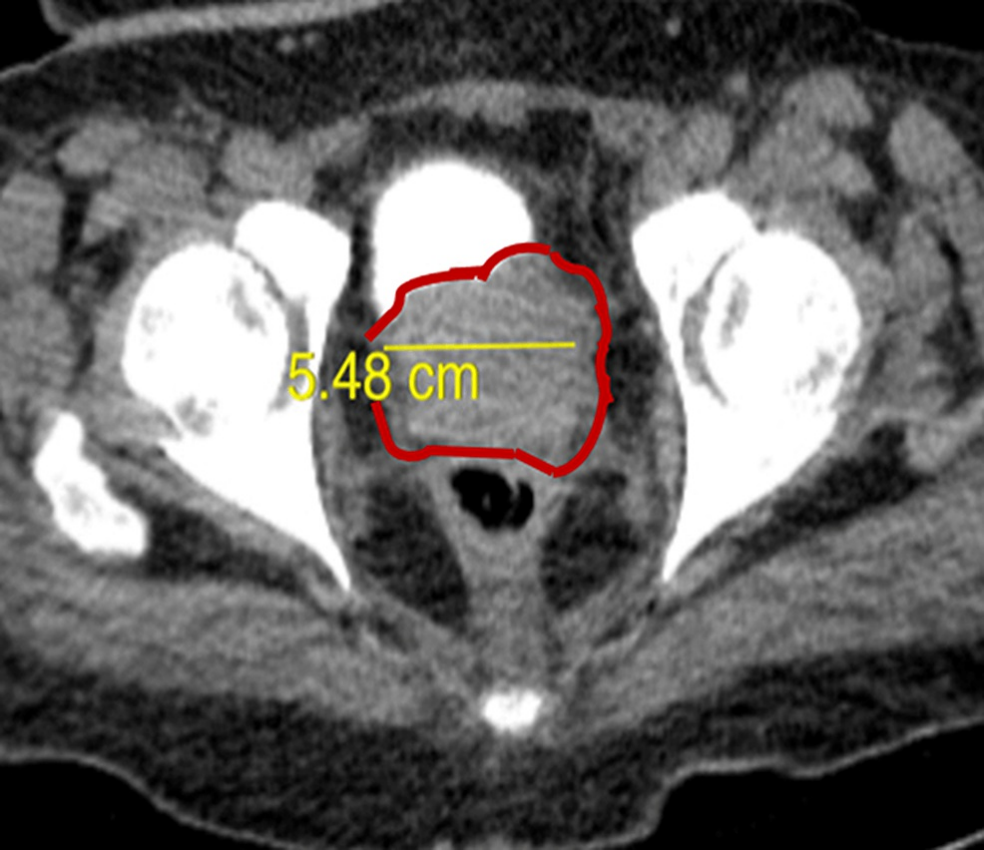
Axial CT scan of the pelvis. Pelvic CT scan showing enlarged prostate measuring 5.48 cm in diameter with indentation along the posterior aspect of the bladder.
